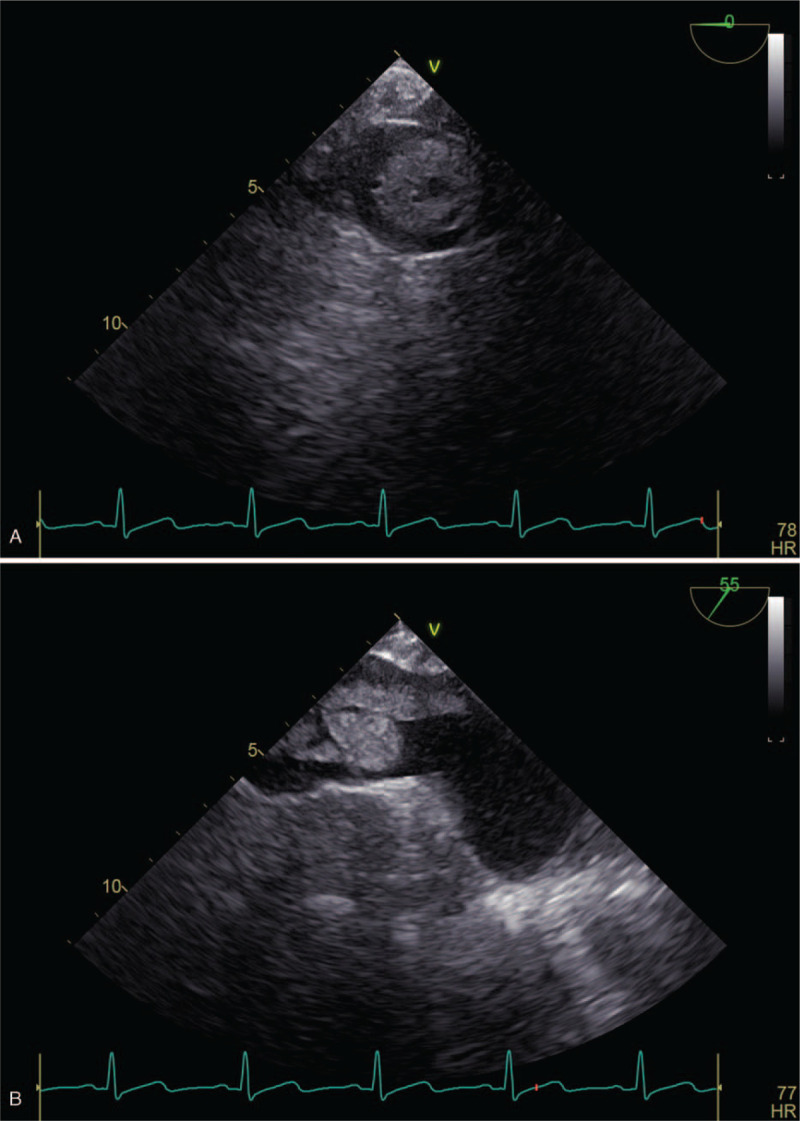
(A) TEE of tumor thrombus. (B) TEE of tumor thrombus. TEE = transesophageal echocardiography.
